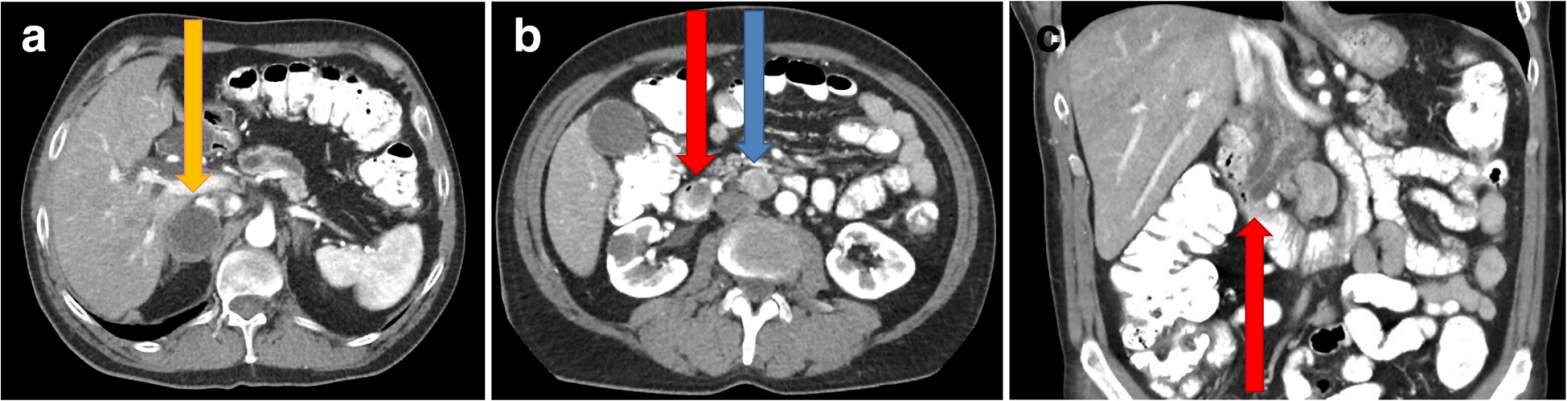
a Abdominal CT (transversal scan) - expansion of the right adrenal gland 4 × 4 cm in size (orange arrow). b Abdominal CT (transversal scan) - tumor of the ampulla of Vater (red arrow) with associated lymphadenopathy (blue arrow). c Abdominal CT (coronal scan) - tumor of the ampulla of Vater (red arrow) with a double-duct sign
